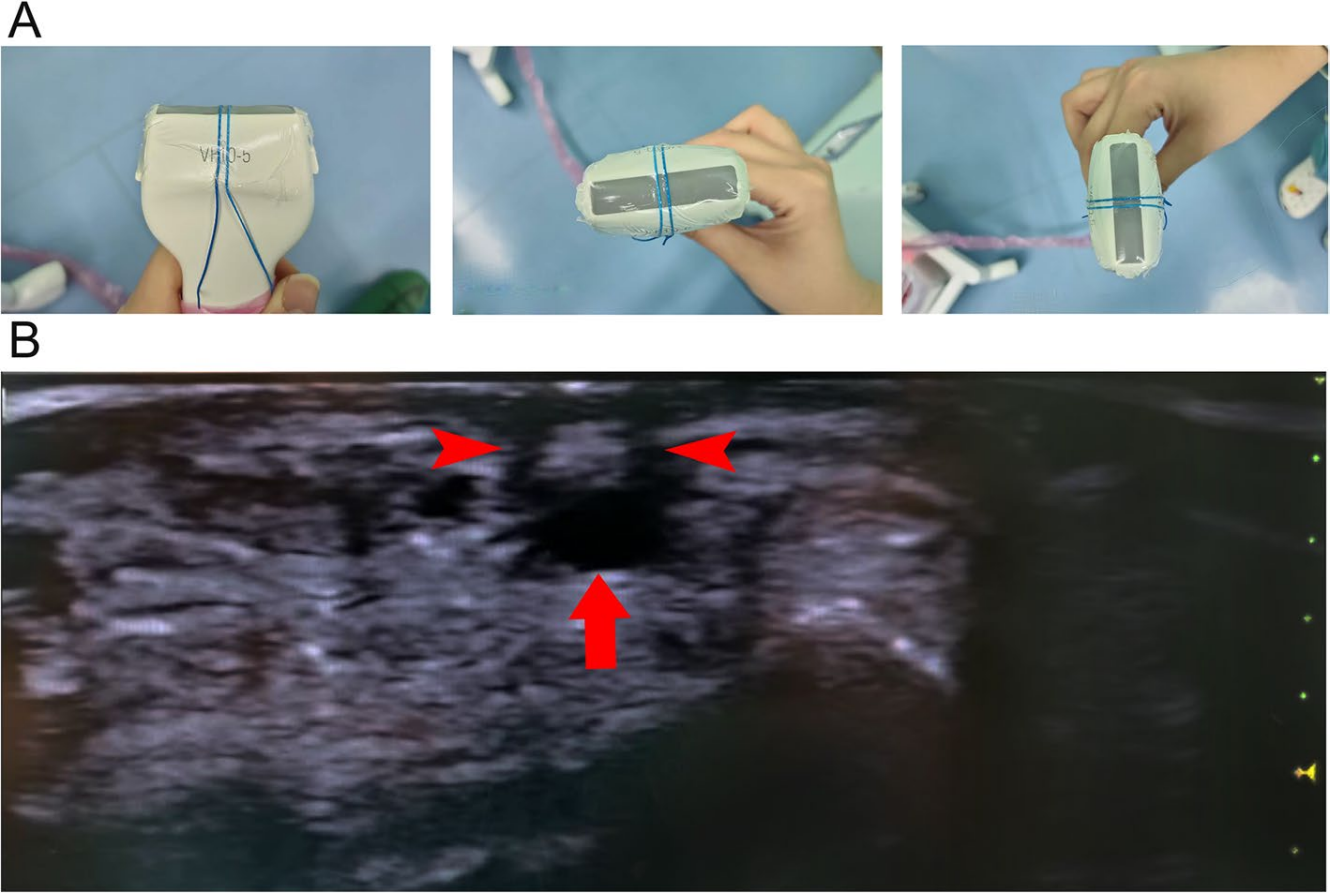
The double developing lines were tied on the ultrasound probe perpendicular to the long axis. A Design of ultrasound probe for double developing lines. B Ultrasonic image of the radial artery (arrow) and the acoustic shadowing of the double developing line (arrowheads)
